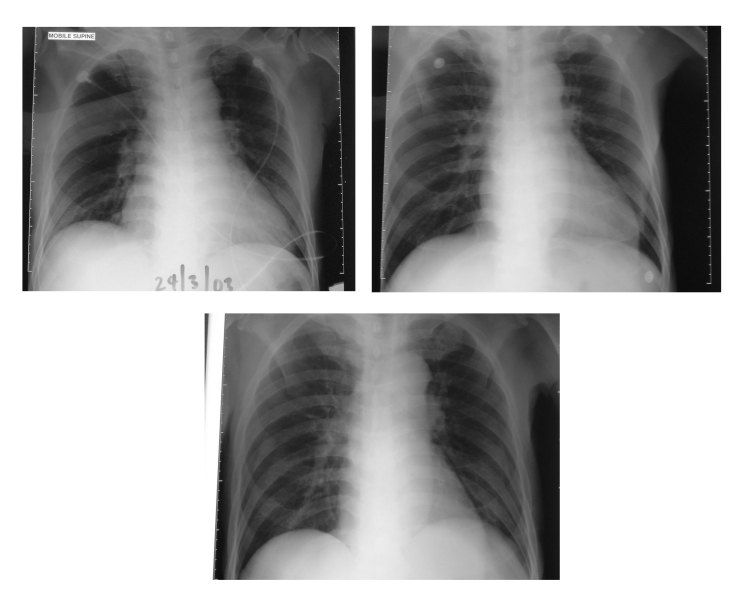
A, radiograph on admission; B, radiograph on day 5 of hospital stay; C, radiograph on day 7 of hospital stay.
